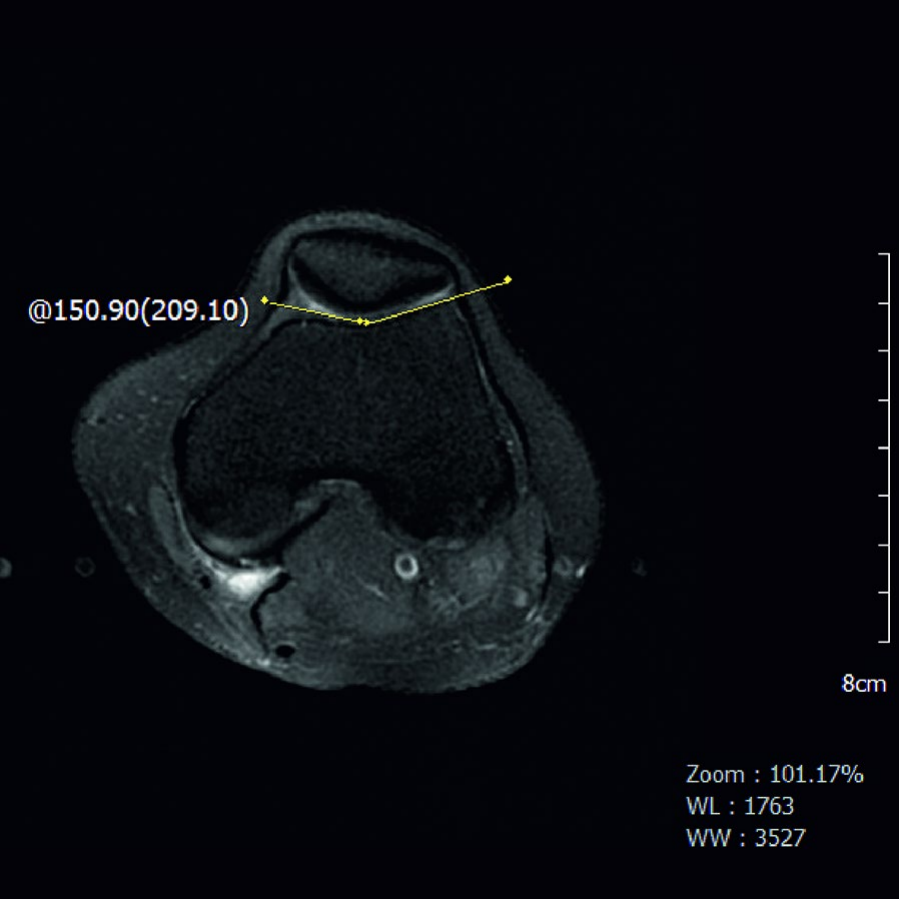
Axial PD-fatsat MRI of a Sect. 3 cm above the knee joint. Although there is type A trochlear dysplasia and the sulcus angle is increased to 150°, the trochlea is symmetric
